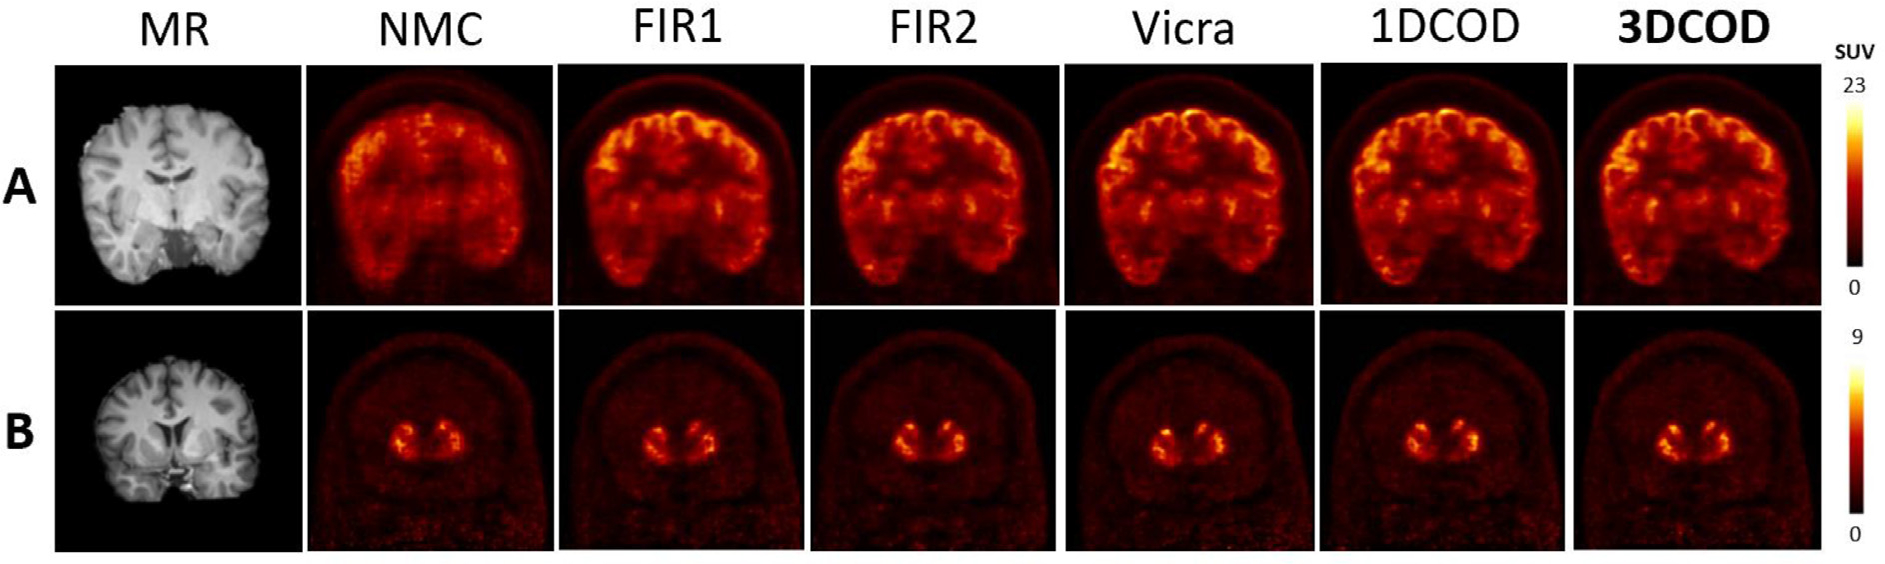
Sample slices of motion-corrected reconstructions of real (A) ^18^F-FDG (60–90 min) and (B) ^11^C-RAC (30–60 min) studies. Studies are ranked 10/10 (worst case) based on mean difference for the 3DCOD-based approach.
